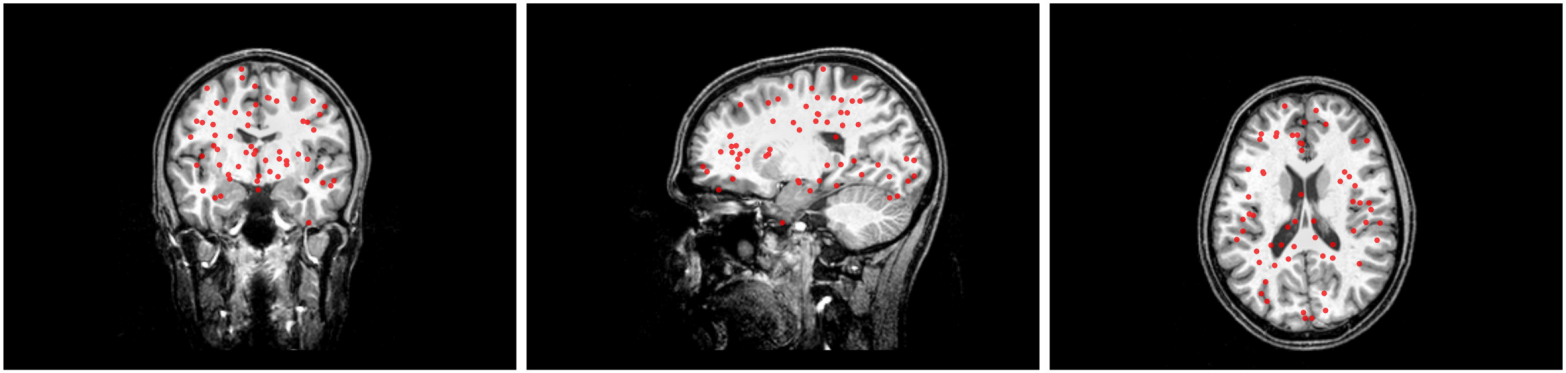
Position of the sources in the brain. Sources as dots, overlayed to a transparent MRI.
